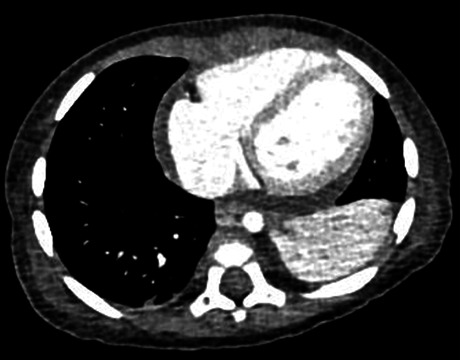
Contrast-enhanced computed tomography scan of the chest showing a pericardial effusion and left partial atelectasis.
